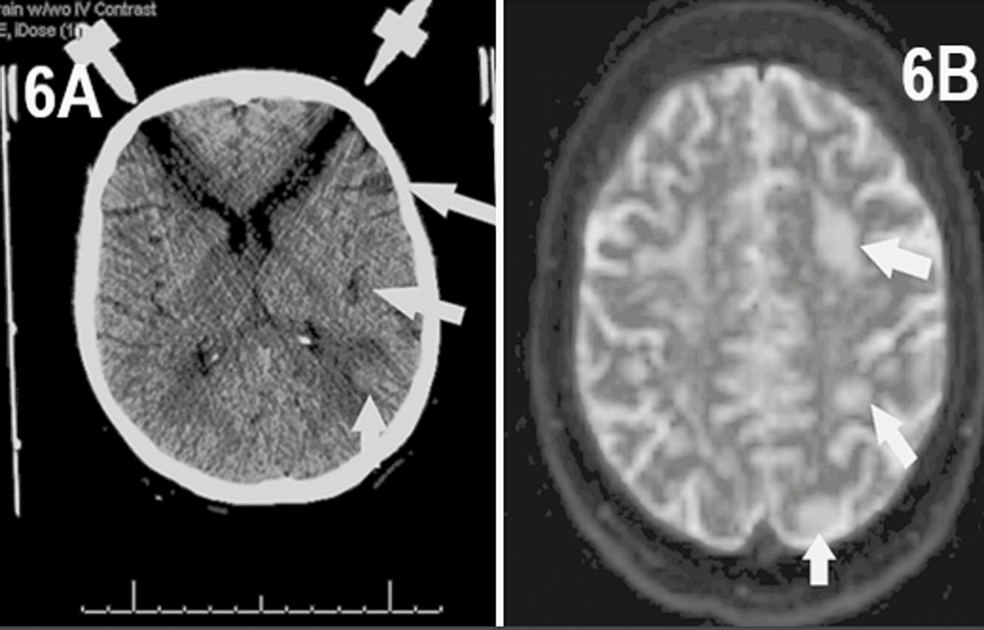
CT Head Conducted One Month After the Identification of Pulmonary Mass (Figure 6A) and MRI Brain Conducted Four Months After Identification (Figure 6B), Both Images Showing the Presence and Progression of Brain Metastases 6A: CT Head conducted one month after the identification of a pulmonary mass. This imaging was conducted to find potential brain metastases after the identification of a pulmonary mass concerning for lung cancer. The imaging identified a hypodense left inferior parietal lobe lesion measuring 1.5*1.1*1.4 cm along with a right inferior enhancing frontal gyrus lesion measuring 1.0*0.9*1.1 cm and an enhancing left superior parietal lobule lesion measuring 1.2*1.0*1.1 cm, confirming the presence of brain metastases and disease progression. 6B: MRI brain conducted four months after the identification of pulmonary mass and three months after the identification of brain metastases. This imaging was conducted to monitor the progression of brain metastases after continued treatment with both SRS and osimertinib. An MRI showed a significant reduction in the size of enhancing metastasis in both the right frontal and left parietal lobe, confirming positive response and improvement after receiving treatment.
